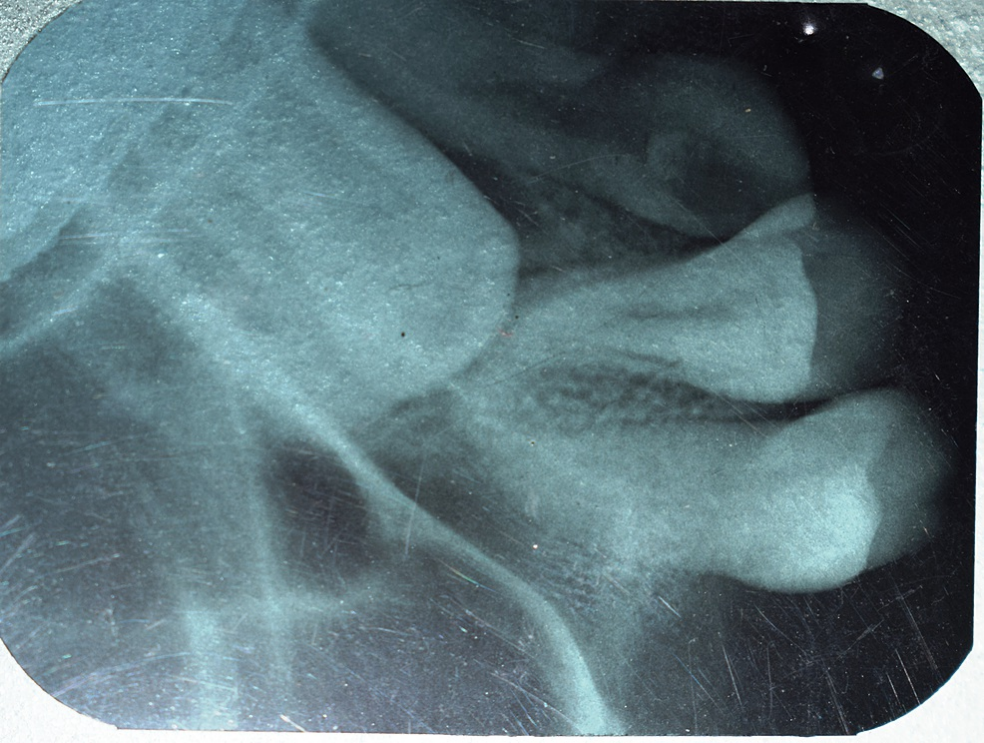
IOPA radiograph with palatally impacted 23 IOPA: intra-oral peri-apical
